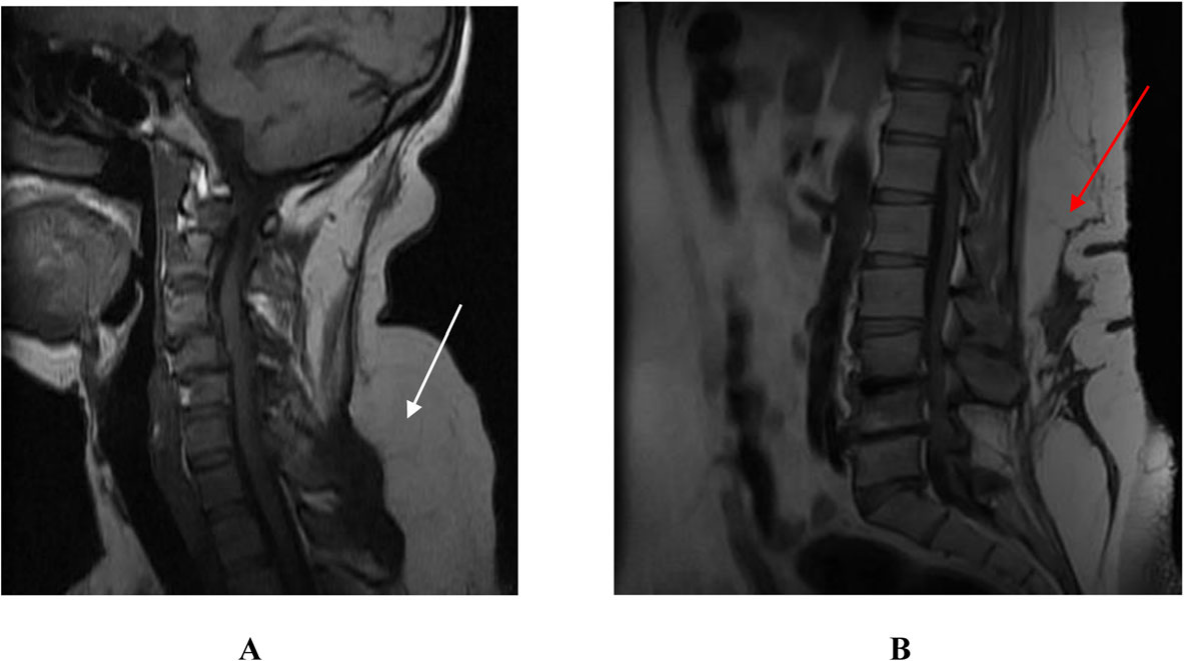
Sagittal T1-Weighted MRI of the cervical (A) and lumbar (B) spine shows huge subcutaneous fat depositions in the posterior neck (white arrow) and the lower back (red arrow) with multilevel degenerative disc disease
